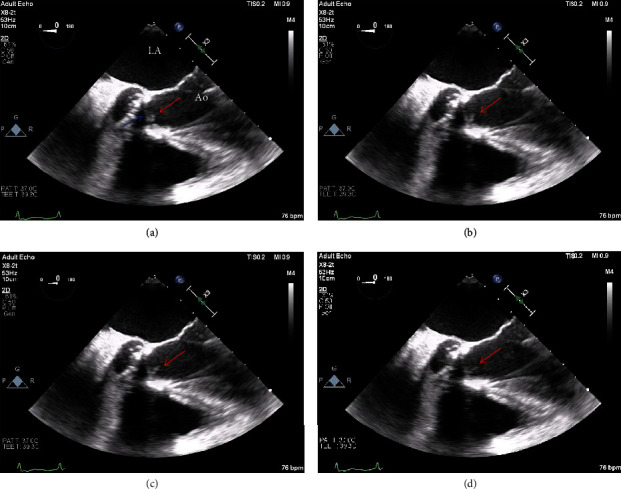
Transesophageal echocardiogram (TEE) midesophageal views (Video 1). Various frames of TEE images showing an elongated and oscillating echogenic structure (red arrow) attached to the right coronary cusp leaflet of the valve-in-valve TAVR (blue arrow) consistent with an infective endocarditis vegetation (measuring 0.7 cm). No significant insufficiency or paravalvular extension of infection was detected. No evidence in favor of valvular thrombosis. The other native valves (especially the mitral valve) had no vegetations. LA: left atrium; Ao: ascending aorta.
